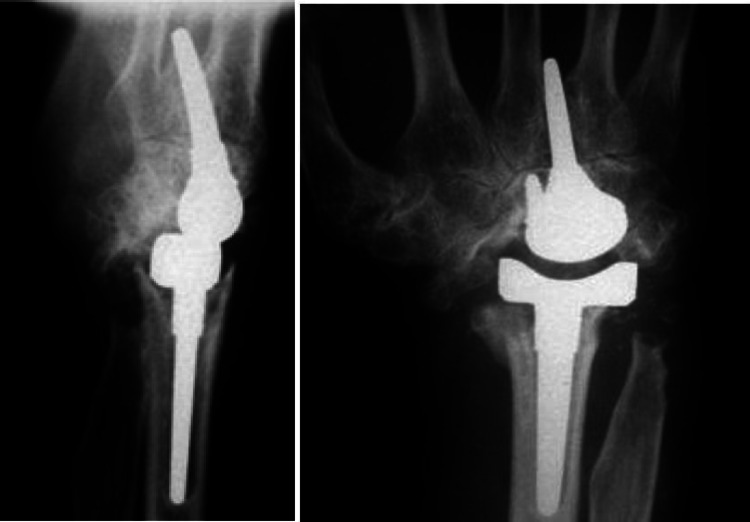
Case 3– Right postoperative X-ray in lateral and a.p. view. Twenty-one months after implantation of a BIAX-prosthesis
